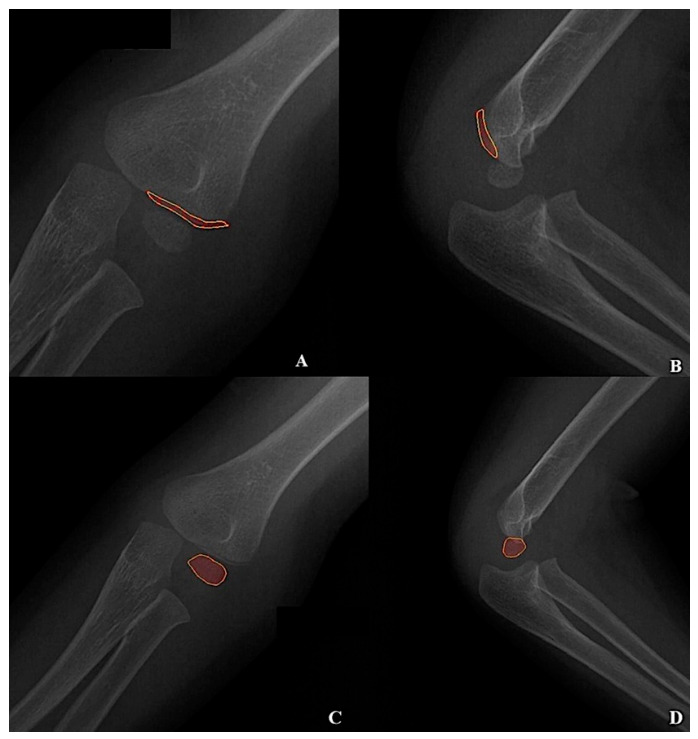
Elbow X-ray. (A). Measurement of fracture fragment area on AP radiograph. (B). Measurement of fracture fragment area on lateral radiograph. (C). Measurement of capitellum area on AP radiograph. (D). Measurement of capitellum area on lateral radiograph.
